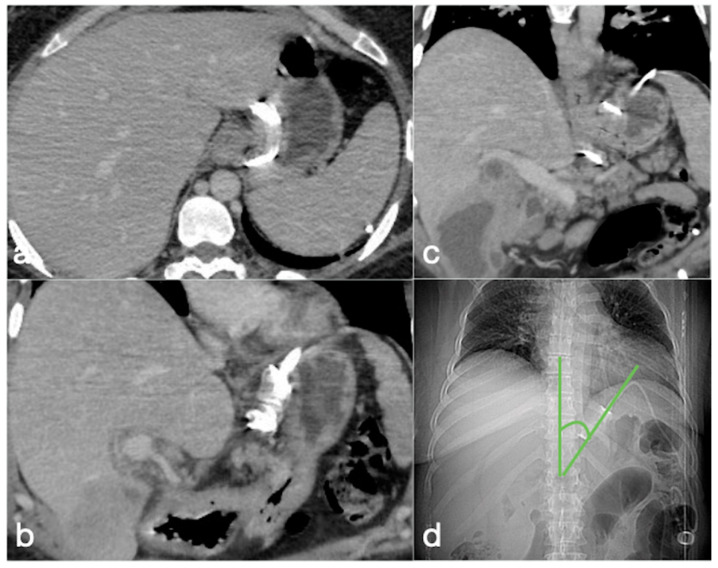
Correct positioning of the gastric banding seen in the CT axial (a) and coronal (b,c) views. (d) The CT localizer at the phi angle. This is the angle that needs to be reviewed to confirm that the gastric band is normally positioned. This is formed by the profile of the gastric band and the vertical axis of the spine on frontal view. The normal range is between 4° and 58°. In the shown case, it is at 40°.
